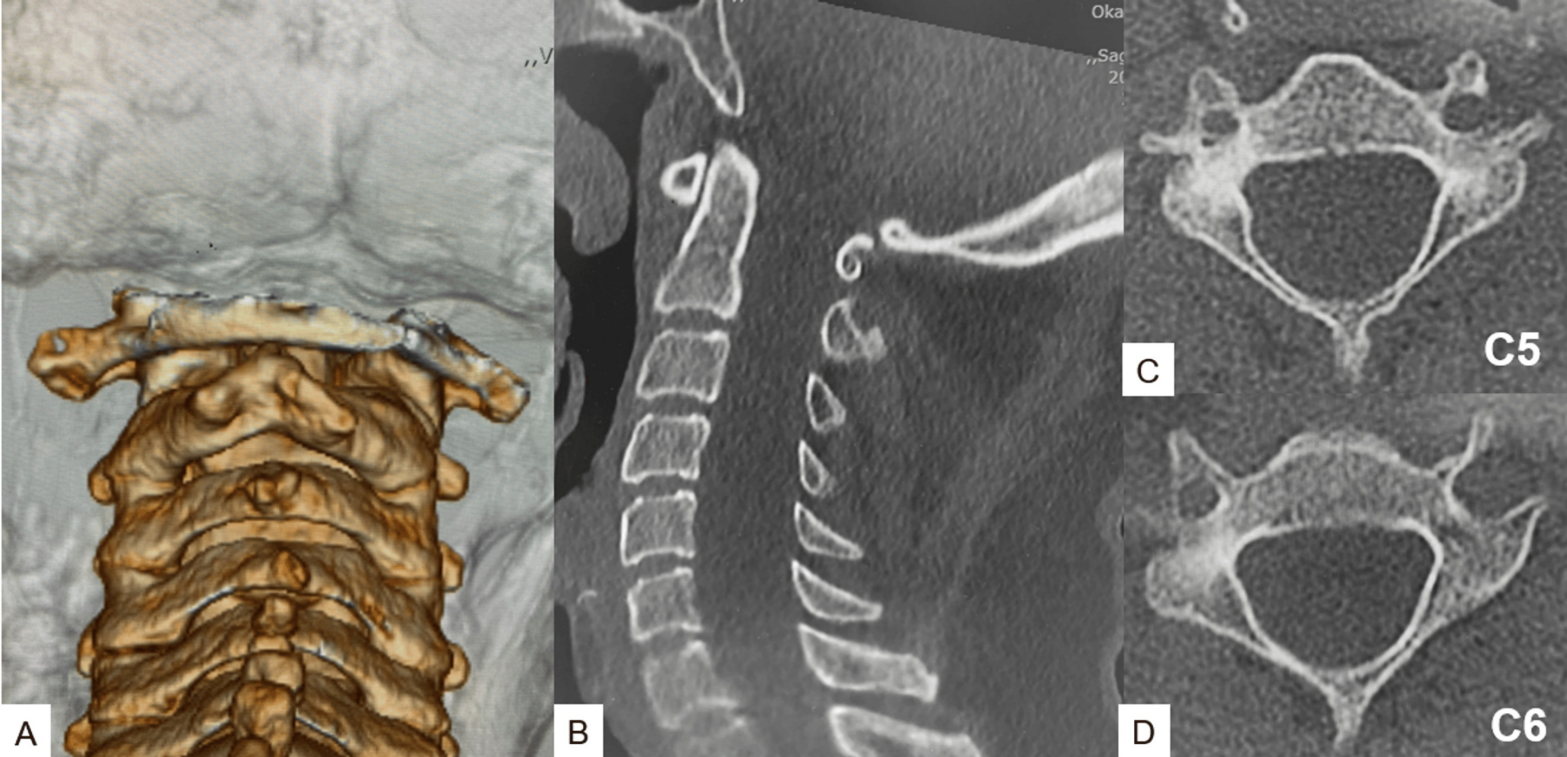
Preoperative CT: (A) posterior aspect of 3D reconstruction CT, (B) mid-sagittal reconstruction, (C) axial section at C5, and (D) axial section at C5. An enlarged spinal canal and bony scalloping can be seen. CT: computed tomography
